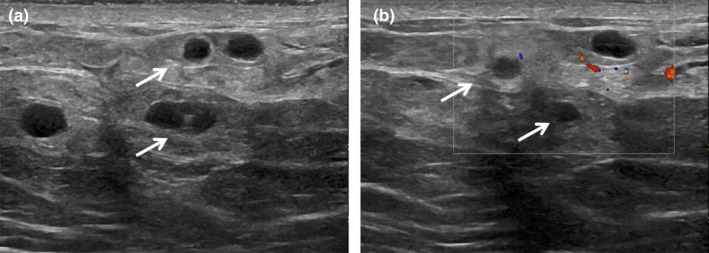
37‐year‐old woman presented to the emergency department with left breast pain. An electronic medical chart review showed a history of breast trauma a month prior. Ultrasound evaluation to exclude a fluid collection carried out by the general on‐call sonographer was performed with a ML6‐15 MHz transducer with the frequency set to 13 MHz. Greyscale (a) and colour doppler (b) images demonstrated multiple round and oval circumscribed masses (arrows), which have the appearance of subacute fat necrosis.
